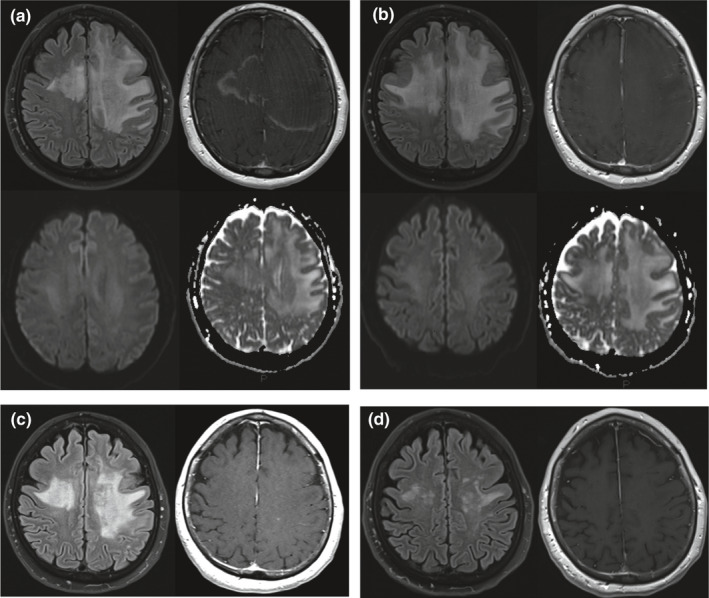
Axial FLAIR and postcontrast T1w images at different time points: additionally, for A and B, DWI trace and ADC sequences are shown. (a) 24 hr after symptoms onset: diffuse hyperintense alteration involving the left frontal lobe and deep white matter of the right frontal lobe, associated with a peripheral ring of contrast enhancement; there is no evidence of restricted diffusion on DWI trace and ADC sequences. (b) 11 days after symptoms onset (after conclusion of intravenous methylprednisolone treatment): disappearing of peripheral ring of contrast enhancement with progression of hyperintense alteration now partially involving the left parietal lobe and, more extensively, the right frontal lobe; there is no evidence of restricted diffusion on DWI trace and ADC sequences. (c) 25 days after symptoms onset (after first cyclophosphamide administration): initial reduction in size of T2 hyperintense alteration, without contrast enhancement. (d) 50 days after symptoms onset (after second cyclophosphamide administration): further reduction in size of T2 hyperintense alteration without contrast enhancement*
